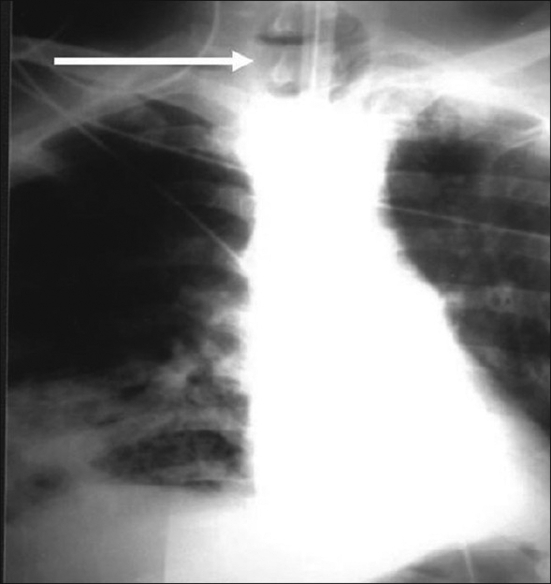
The arrow in chest radiograph shows tracheoesophageal fistula
